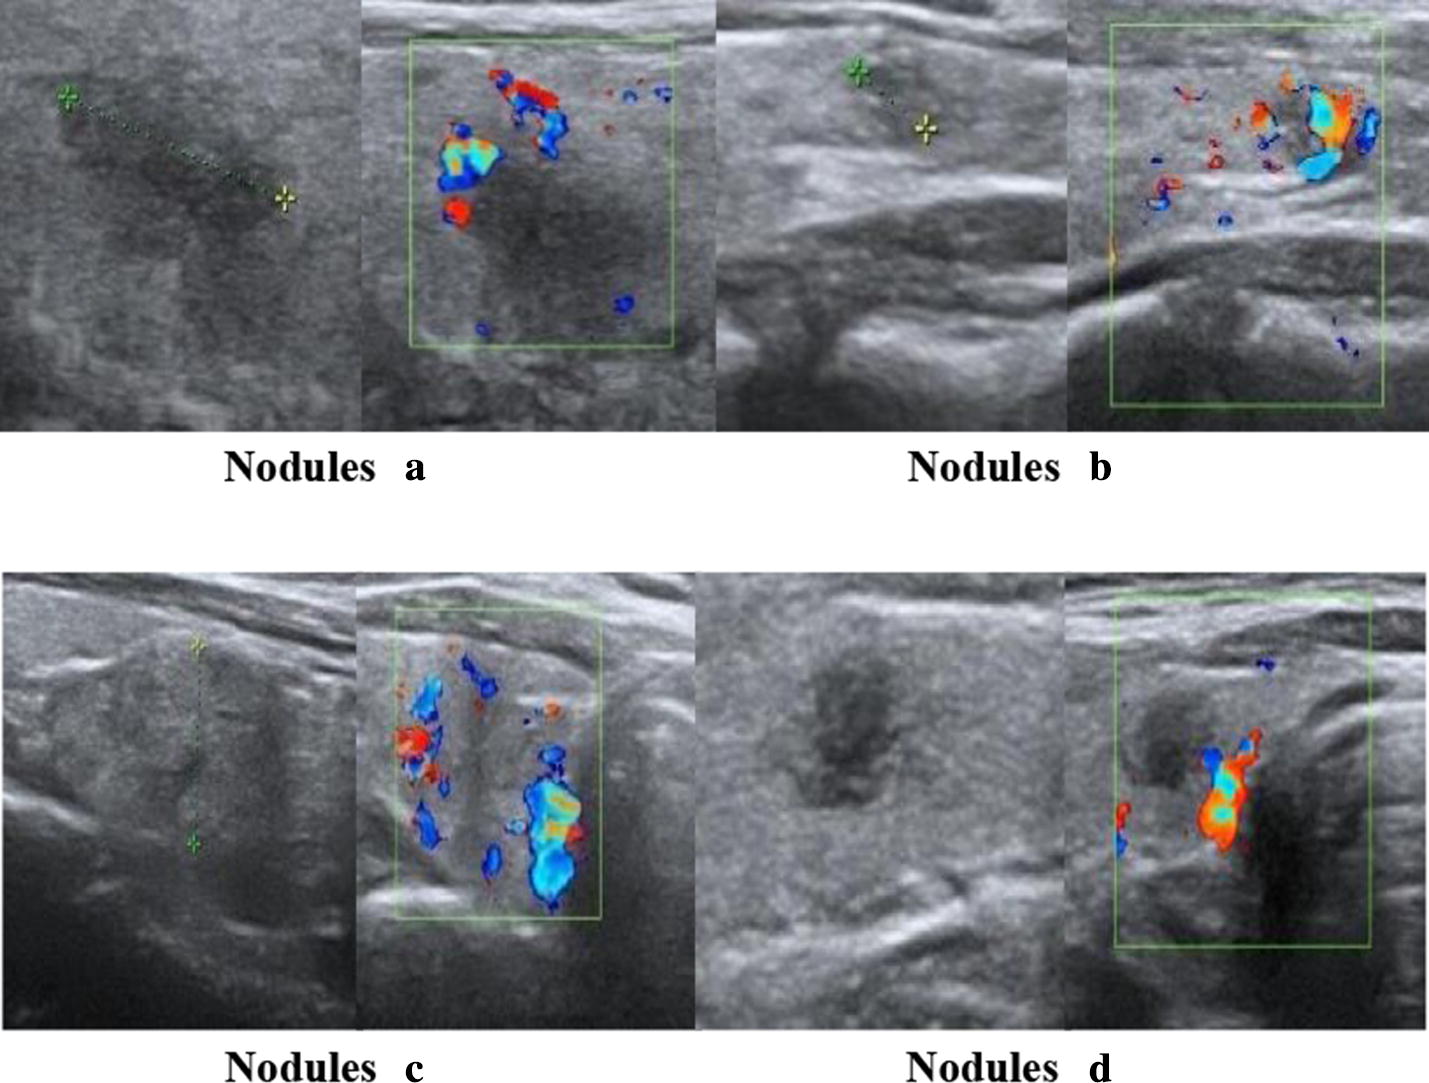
Examples of wrong classification in our TIRADS. Four thyroid nodule samples which classification results are inconsistent with radiologists, named nodules (a–d). Each sample includes a grayscale ultrasound image and corresponding Doppler color ultrasound image
